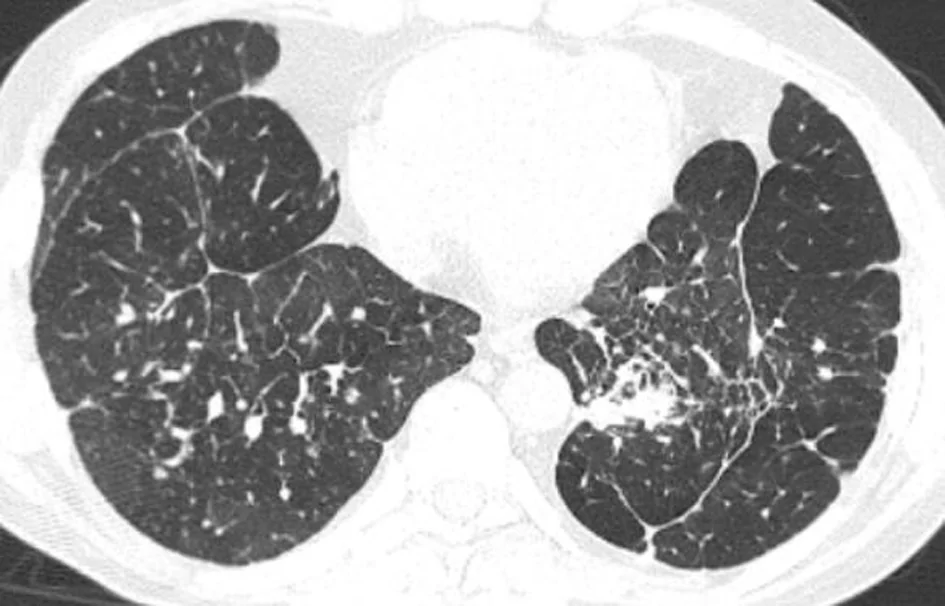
Axial HRCT image (lung window) demonstrating diffuse interstitial reticular changes with interlobular septal thickening, nodular thickening of bronchovascular bundles, and ill-defined ground-glass opacities with preserved bronchial patency. HRCT: high-resolution computed tomography.
